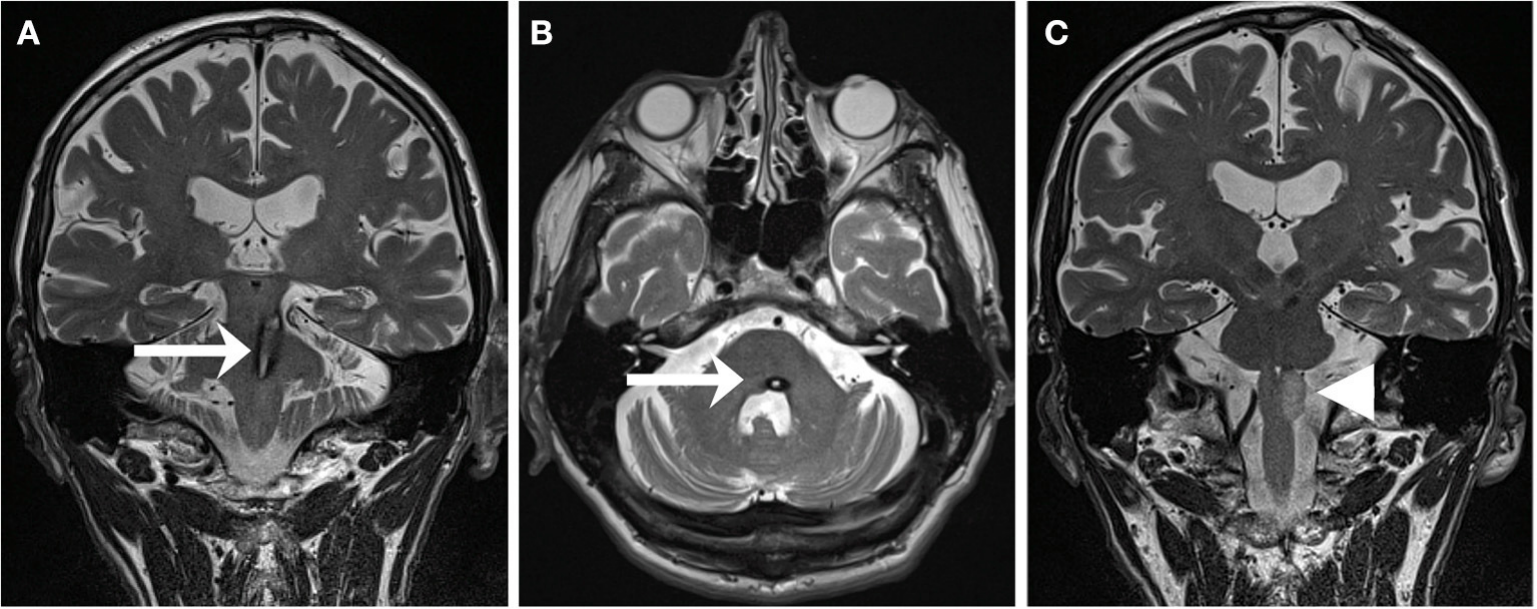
T2-weighted MRI of a 59-year-old patient suffering from pontine-mesencephalic bleeding (A,B) affecting the central tegmental tract on the left (arrows). Within 13 months, the patient developed a HOD (C) with hyperintensity of the left olive (arrowhead) accompanied by the clinical syndrome of a palatal tremor, a pendular nystagmus, and a Holmes tremor [adapted with permission from Foerch et al. (10)].
